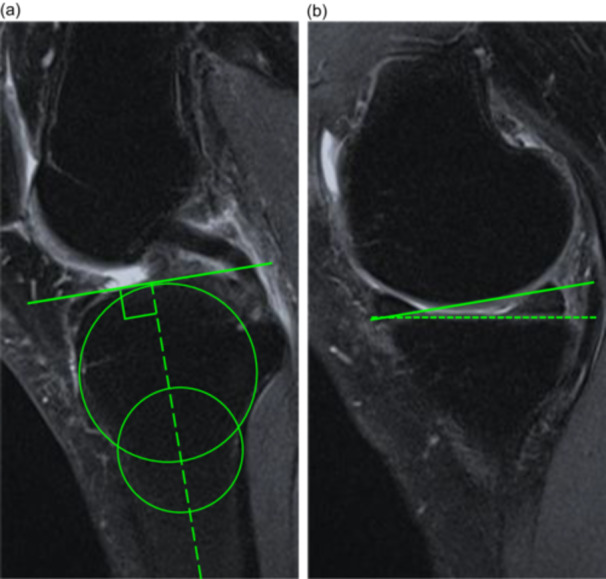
Magnetic resonance image of the posterior tibial slope. (a) On the sagittal plane in which the centre of the posterior cruciate ligament is visualized, two circles are drawn, each tangent to the cortical margins of the bone. The line connecting the centres of these circles is defined as the tibial axis (long dashed line). The solid line is perpendicular to the tibial axis. (b) On the sagittal plane in which the posterior slope of the medial tibial plateau is visualized, the posterior tibial slope is measured as the angle between the solid line and the dashed line connecting the most superior anterior and posterior cortical edges.
